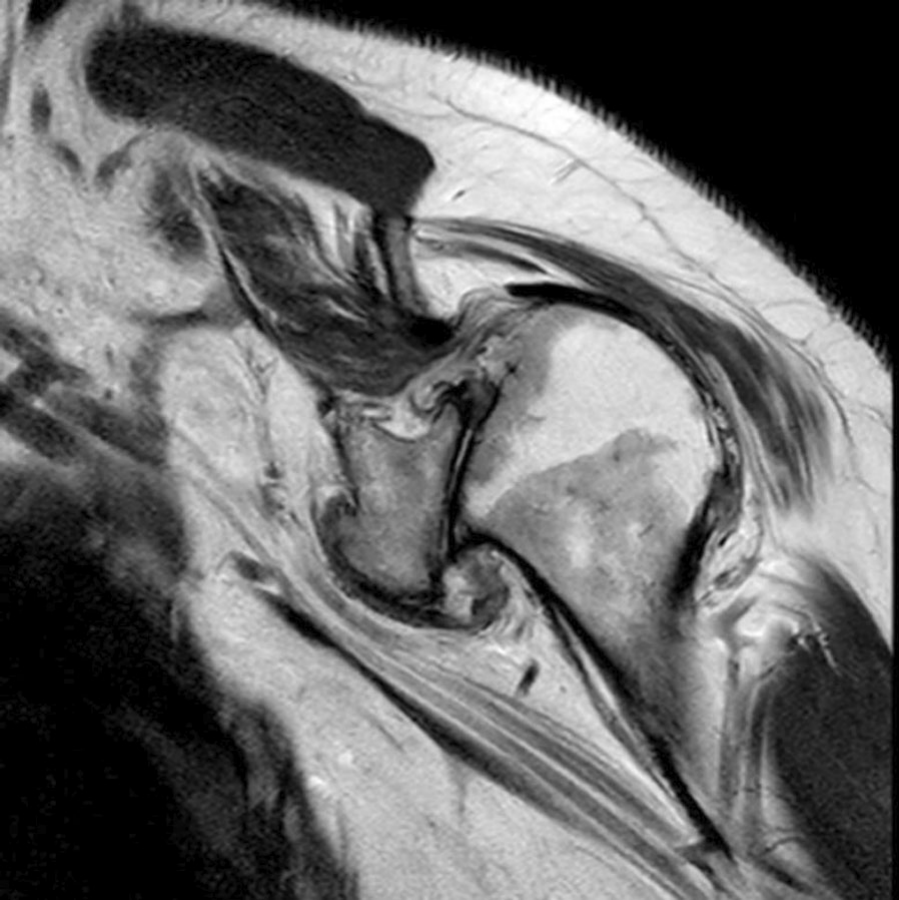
T2W sagittal plane MRI showing massive rotator cuff tear with abnormal arthritic erosion of humeral head
